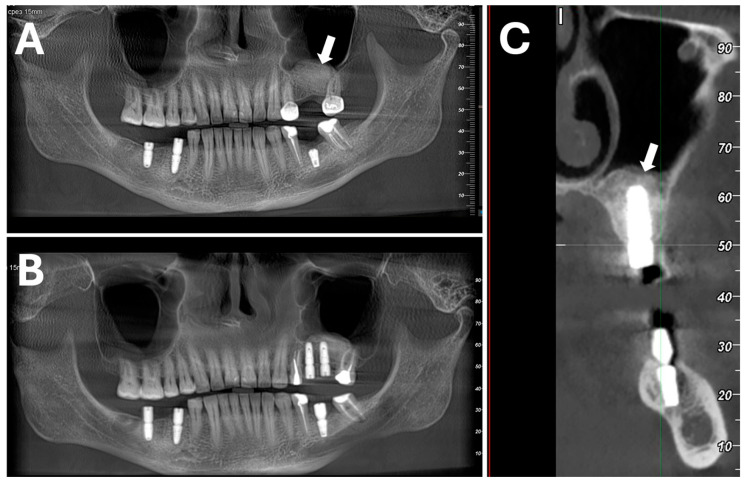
Use of Ivory Dentin Graft^TM^ for unilateral sinus augmentation. Status at 5 m when implants inserted. Radiographs show: (A) Left-side sinus wall augmentation (arrow); (B) implants inserted into augmented maxilla; (C) implant is well covered and supported by augmented bone (arrow). Unpublished case from Dr Sapoznikov of 72-year-old female.
